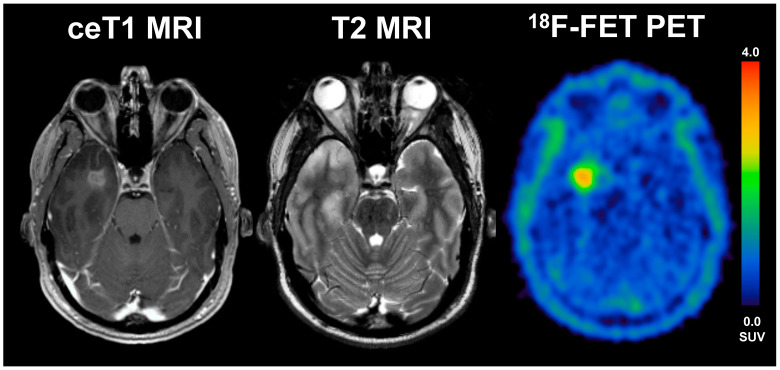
A 33-year old male patient with glioblastoma (WHO grade IV, IDH wildtype, *MGMT* methylated) and an overall survival of 47 months (LTS). The diameter of CE on T1 MRI was 17 mm, volume of CE was 28 mL, the BTV in FET-PET was 16 mL, the TTP_min_ was 17.5 min and TBR_max_ 4.1. The patient underwent radio-chemotherapy.
